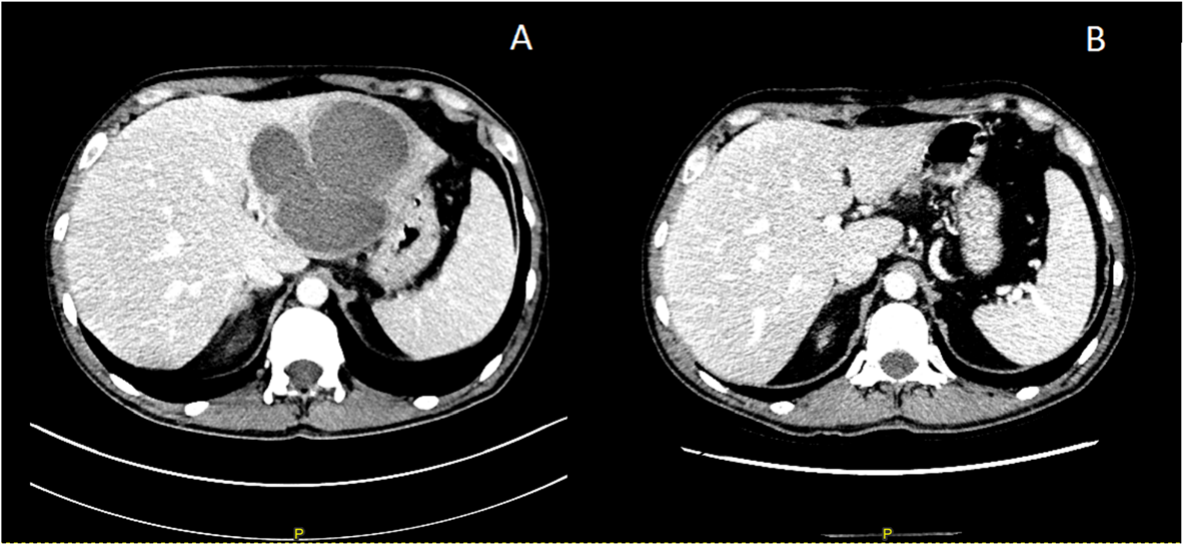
Panel a: CT-scan showing enlarged left liver lobe due to the presence of a single large loculated abscess cavity. Panel b: CT-scan performed 3 months later showing a normal liver structure
